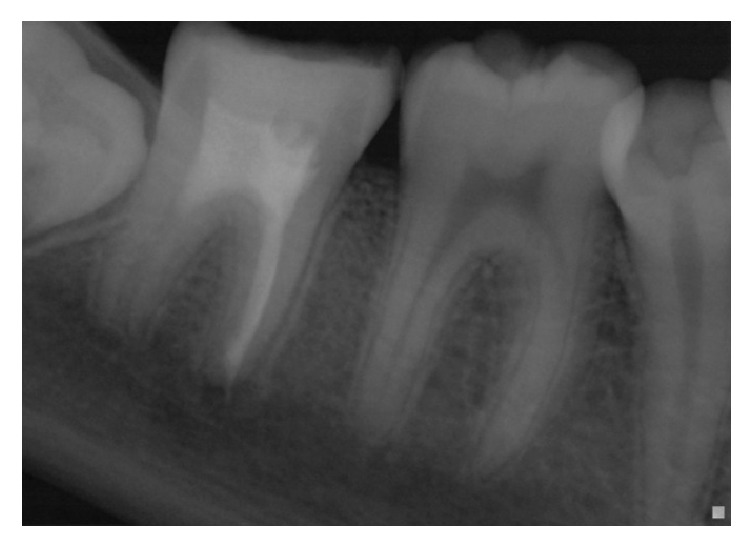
Radiograph at 24 months' recall: complete disappearance of the radiolucent images.
